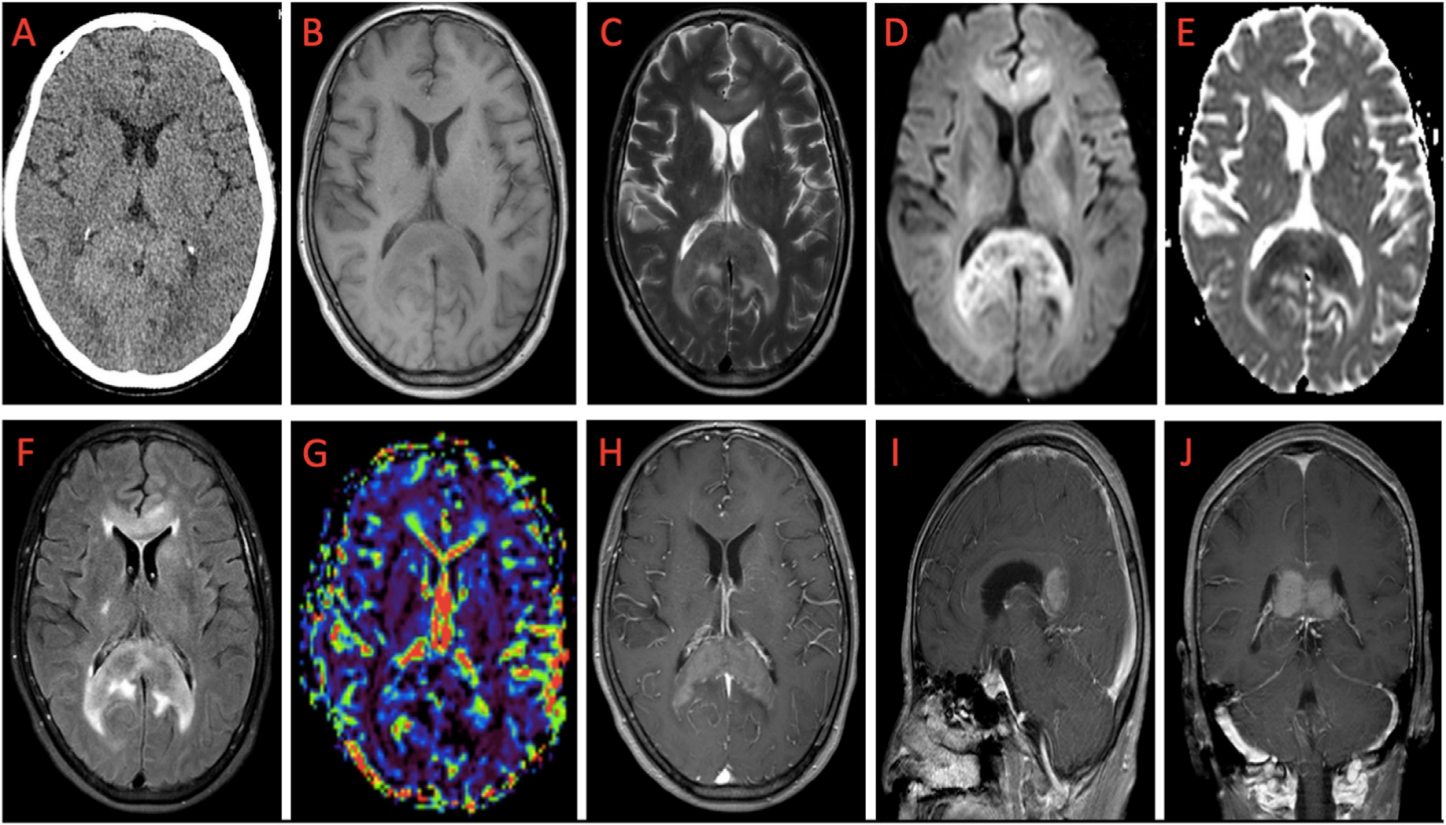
Multimodal imaging findings of a patient with primary CNS lymphoma in the splenium of the corpus callosum: A: slightly higher CT plain scan density; B: hypointense in T1; C: hypointense in T2; D: hyperintense in DWI; E: hypointense in ADC; F: hyperintense in FLAIR; G: hypoperfusion in Perfusion; H–J: Pterygoid, symmetrically distributed, heterogeneous enhancement was seen on axial, sagittal and coronal T1 enhancement.
